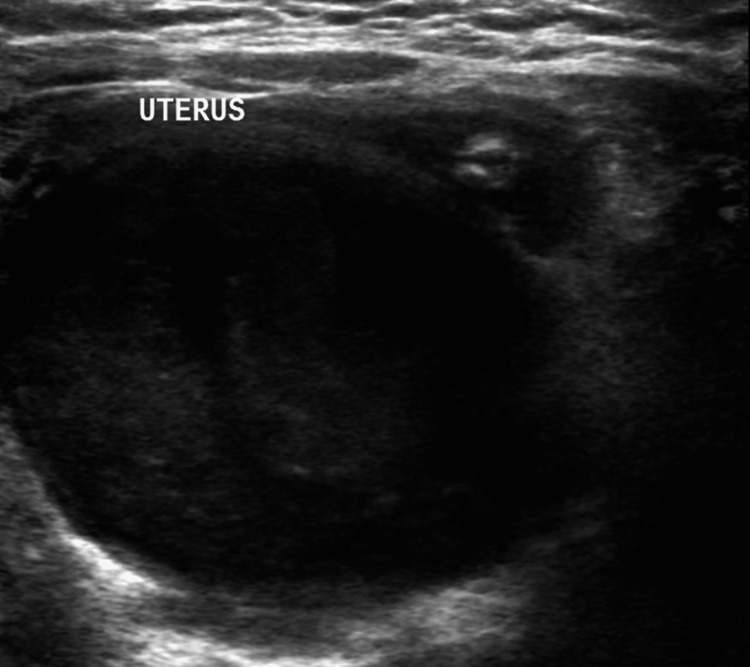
Grayscale ultrasound of the abdomen and pelvis showing a large retrovesical cyst containing turbid fluid.
